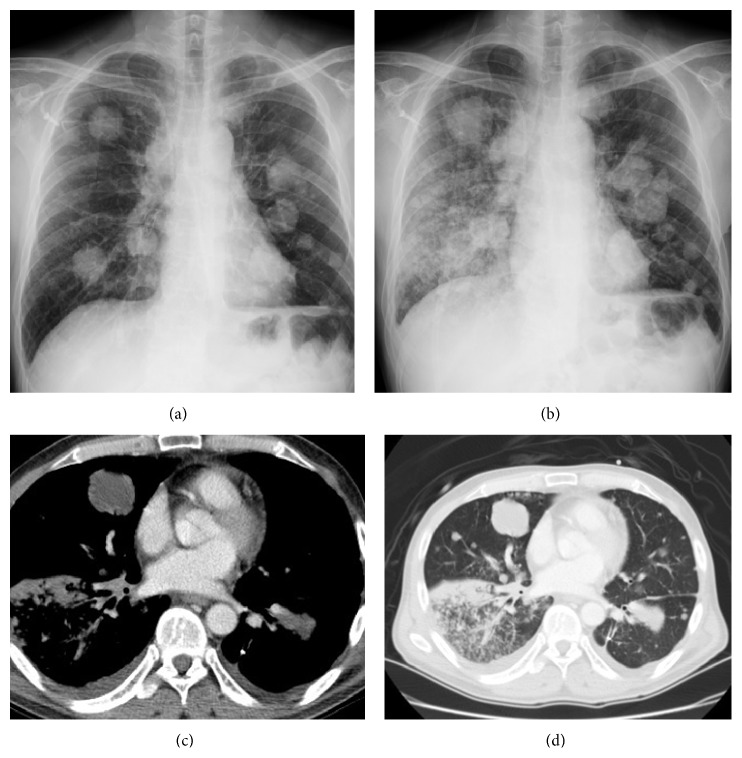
Pulmonary vein obstructive syndrome (PVOS). A 54-year-old man with bladder cancer. Chest X-ray (a) before and (b) at the onset of PVOS showed right low lung and hilum increase haziness. CT scan ((c) and (d)) revealed tumor/thrombosis located in right inferior pulmonary veins with peripheral extension.
